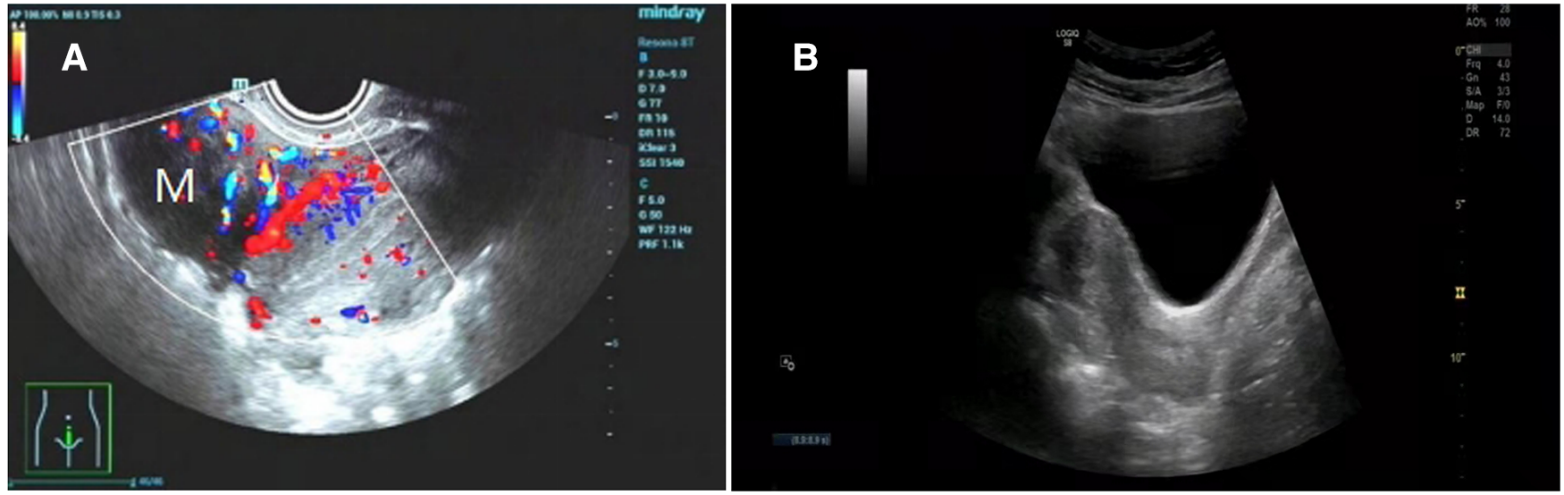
(A) Preoperative ultrasound showed multiple uterine leiomyomas. (B) Ultrasound image of normal uterus 6 months after surgery.
